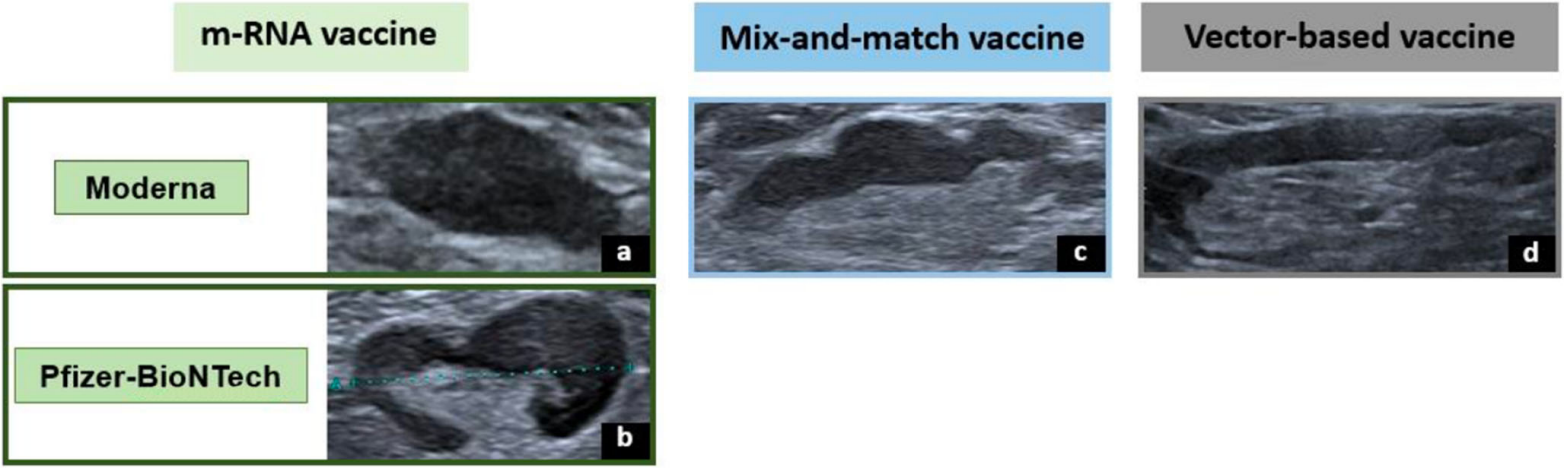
Comparative lymph node morphology in imaging ultrasound from vaccine recipients with different vaccination protocols. a A type Bedi 6 node with an absent hilum seen in a volunteer vaccinated with Moderna protocol. b A type Bedi 5 node with a focal hypoechoic cortical lobulation detected in a volunteer vaccinated with Pfizer protocol. c A type Bedi 4 node with a generalized lobulated cortex seen in a volunteer after receiving the mix-and-match protocol. d A type Bedi 3 node with a diffuse regular hypoechoic cortex greater than 3 mm in a volunteer vaccinated with full AstraZeneca protocol
